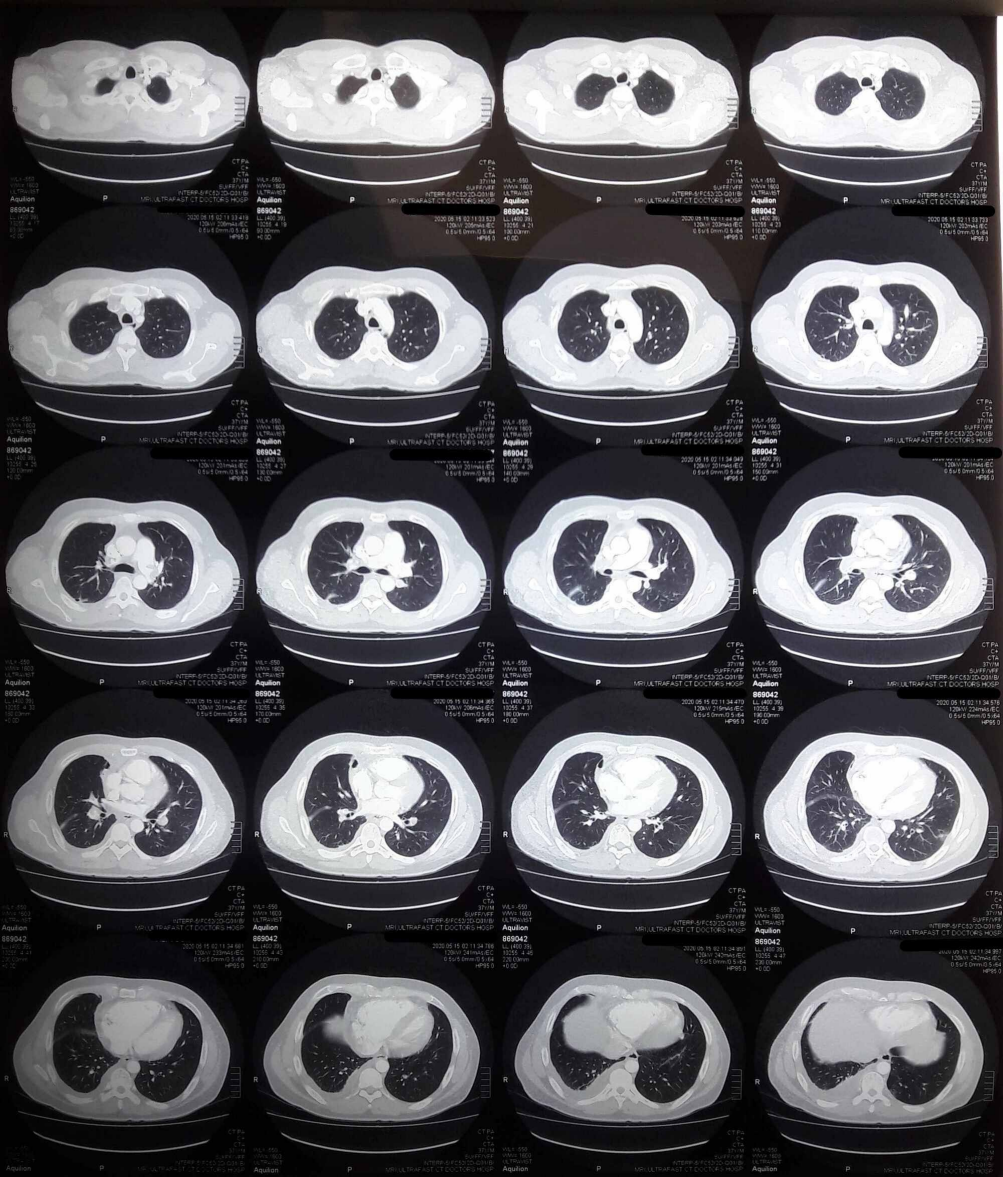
Chest high-resolution computed tomography (axial plane). Large right pleural effusion and infiltrates in the left lung.
